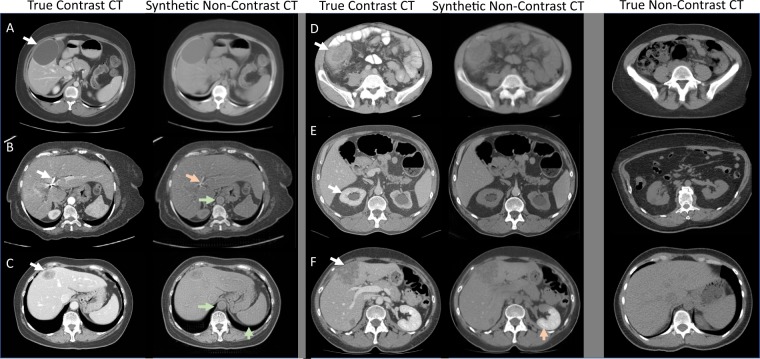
Examples of true IV contrast CT scans (left column) and synthetic non-contrast CT scans generated by a CycleGAN. The rightmost column shows unrelated example non-contrast images. Overall the synthetic non-contrast images appear convincing - even when significant abnormalities are present in the contrast CT scans.
